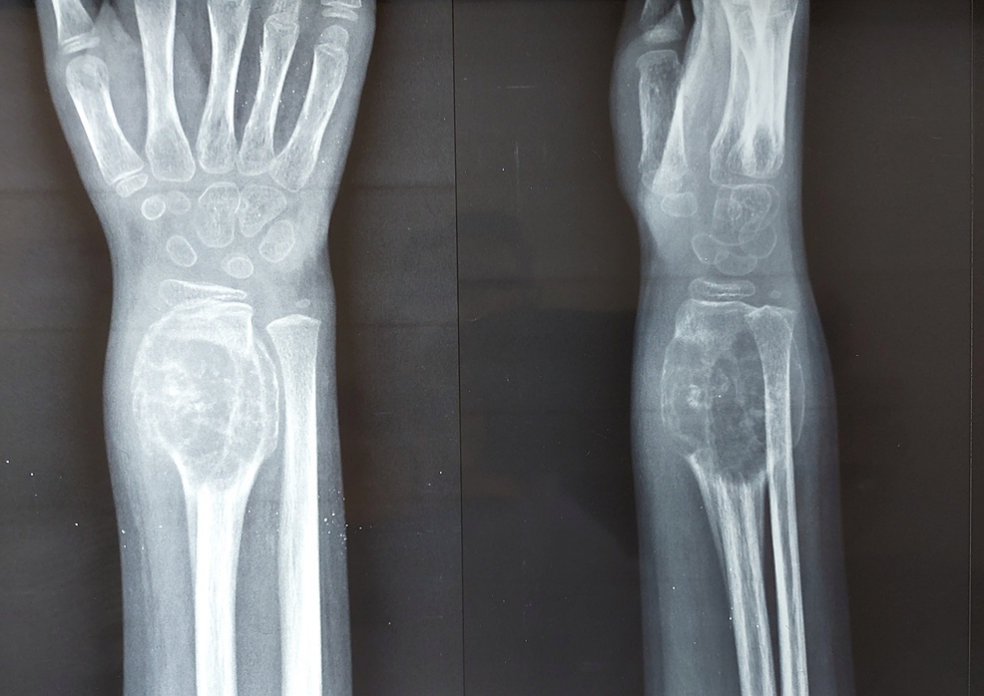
An X-ray at presentation showing an expansile lytic lesion at the distal end of the radius
